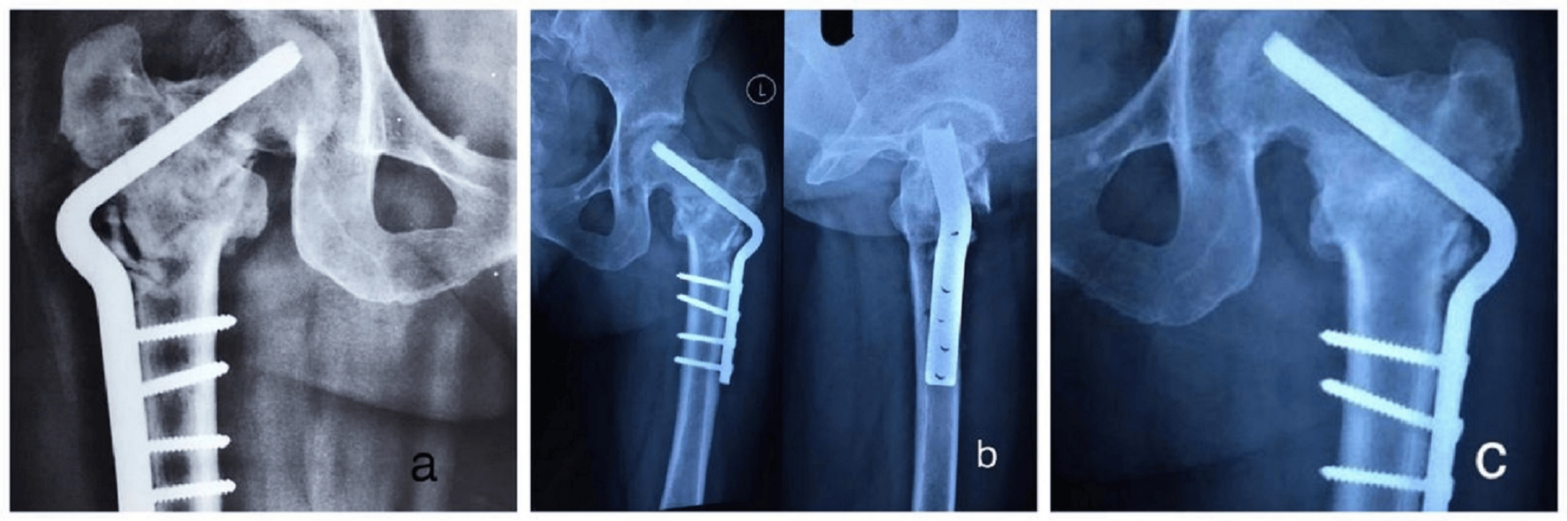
Follow-up radiographs for DABP patient. (a) Immediate postoperative X-ray;
